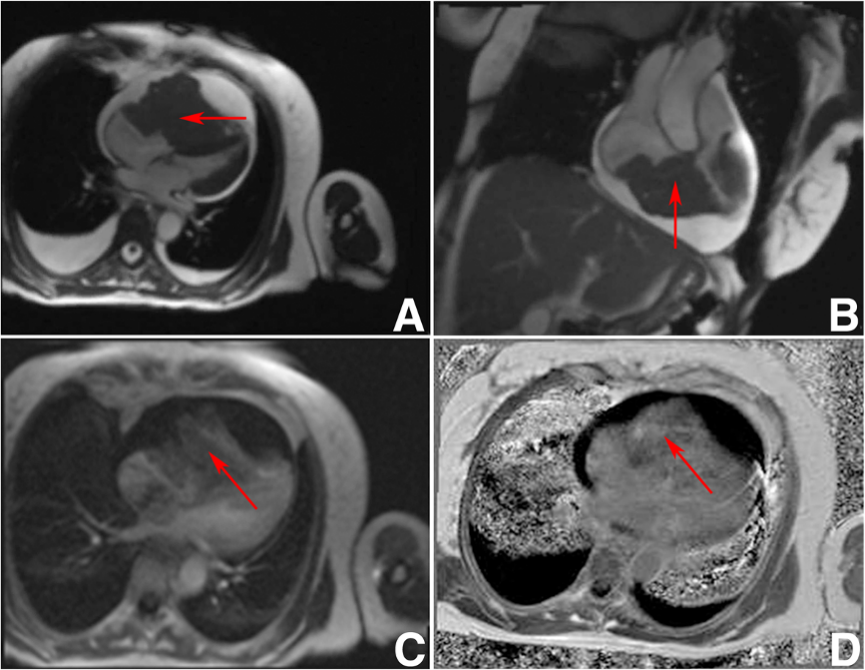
Preoperative patient 2 Heart MRI. a 4 chambers GRE view. Hypointensive masses visible in right ventricle, right atrium and right atrial appendage (red arrow). The tumour is obstructing the right ventricle and compressing the interventricular septum, and the left ventricle. b 2 chambers GRE view. Hypointensive masses in the right ventricle and right atrium (*red arrow*). c 4 chambers view perfusion sequence. Perfusion is seen in the masses (*red arrow*). d 4 chambers view. Non homogenous gadolinium enhancement is visible (*red arrow*). All images were taken during diastole
